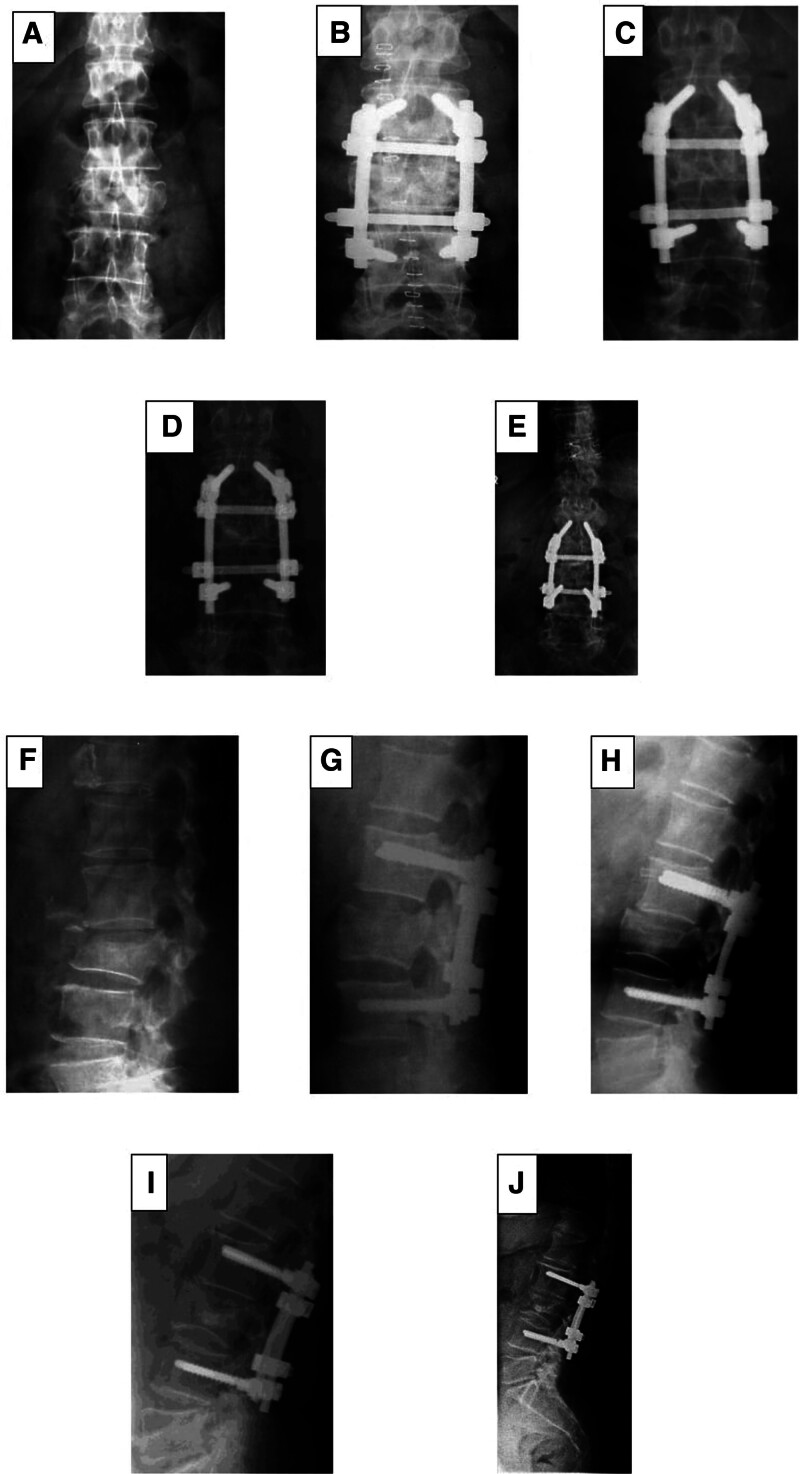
Patient X-ray samples: preoperative AP (A), early postoperative AP (B), 2004 AP (C), 2010 AP (D), 2021 AP (E), preoperative lateral (F), early postoperative lateral (G), 2004 lateral (H), 2010 lateral (I), and 2021 lateral (J). AP = anteroposterior.
